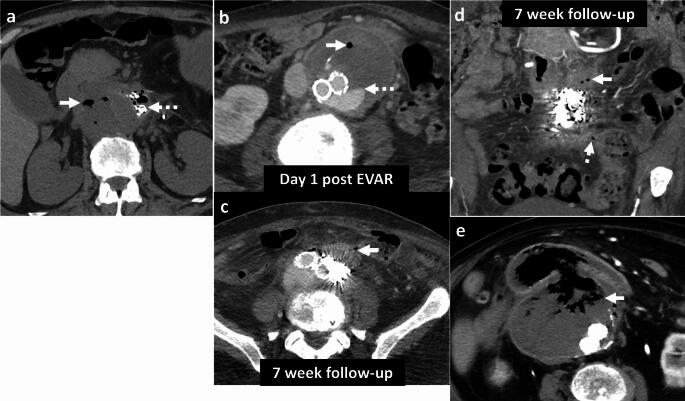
Pitfalls for emphysematous aortitis. a Immediate post-surgical changes. A 73-year-old patient status post-infected stent graft explant with placement of antibiotic beads. Immediate post-operative non-contrast CT a shows expected peri-aortic fluid and air foci (solid white arrow, a) and antibiotic beads (dotted white arrow, a). These findings were resolved on follow-up CT. b–d Immediate post-EVAR changes followed by fistula. An 82-year-old patient status post EVAR with aorto-iliac stent graft. Day-1 post-EVAR CT b shows an expected small speck of air (solid white arrow, b) in the excluded aneurysm sac. There was a type II endoleak (dotted white arrow, b) treated with coil embolization. At 7-week follow-up CT c, d, there was decreased size of the excluded aneurysm sac but increased peri-aortic air foci (solid white arrow, c) and a fistulous connection to colon (dotted white arrow, d). The aorto-colonic fistula was confirmed on surgery. The patient underwent stent explant, aortic repair, colectomy and right colostomy. e Aorto-duodenal fistula. An 86-year-old post-EVAR and aorto-iliac stent graft, with severe abdominal pain and hematemesis. CT shows direct communication between the excluded aneurysm sac and transverse duodenum (solid white arrow, e)
